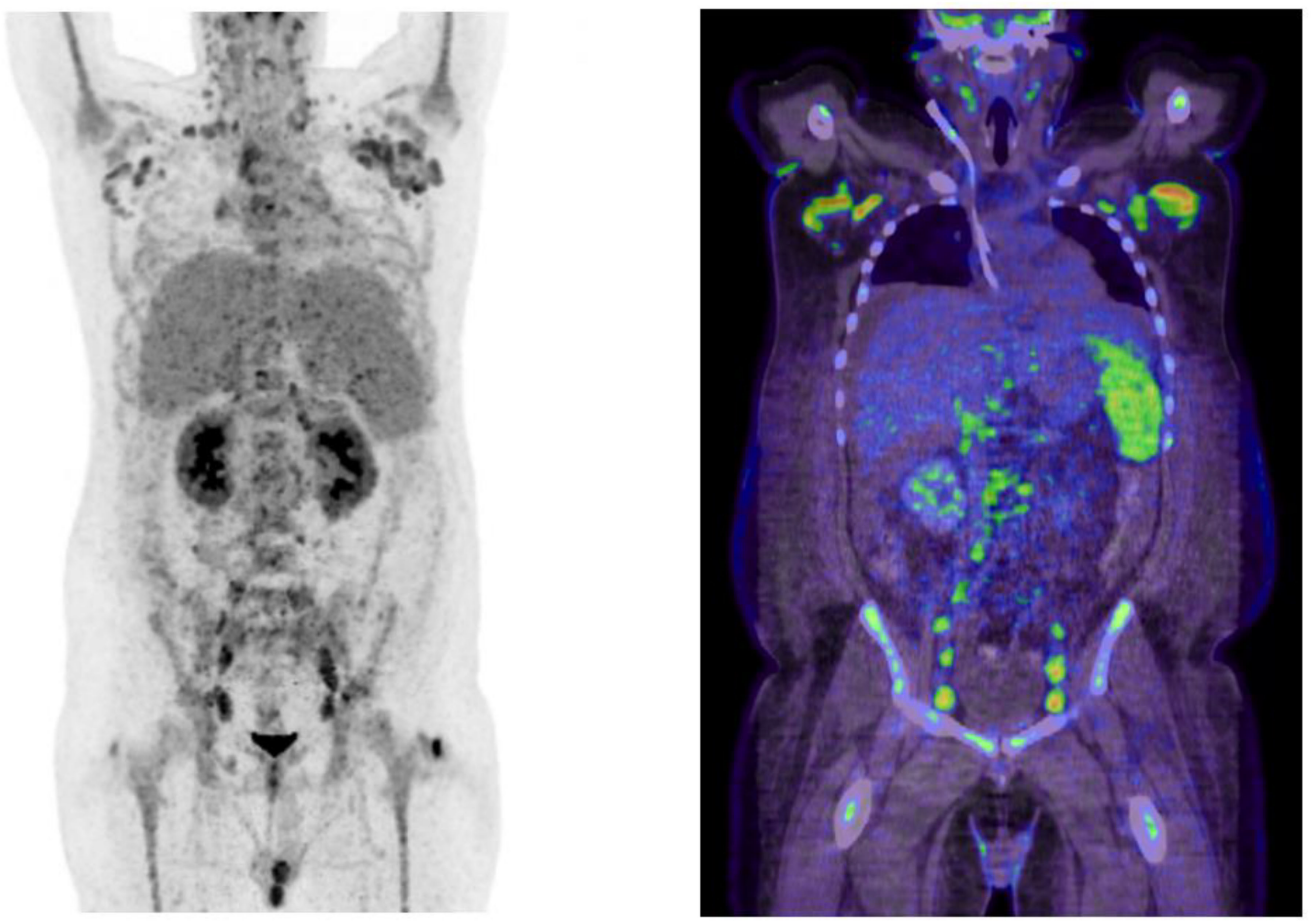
PET CT imaging revealed generalized hypermetabolic lymphadenopathy involving cervical, mediastinal, axillary, abdominal, and inguinal lymph nodes, consistent with multicentric Castleman disease.
